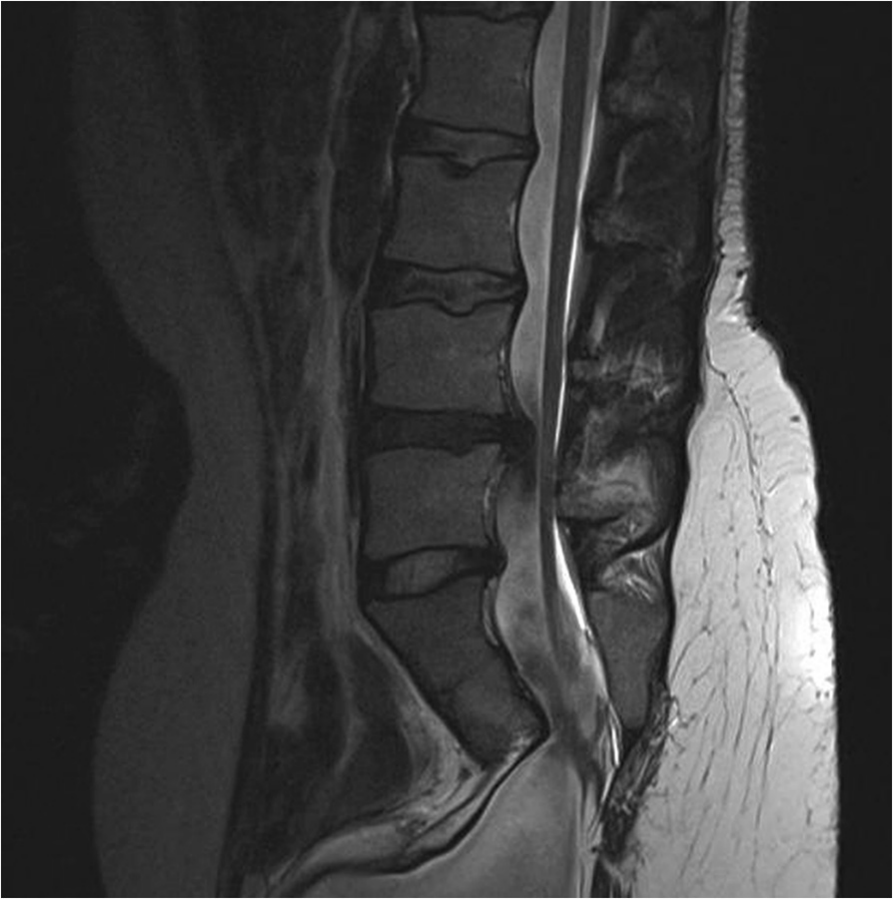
On the MRI image tethering of the spinal cord was seen at L5-S1 level. Additonally a herniation of an intervertebral disc is seen (asymptomatic and left untouched at surgery).
